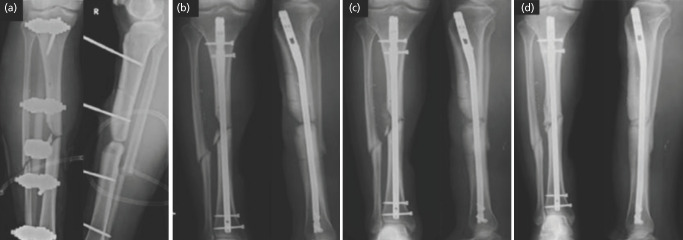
(a) Clinical radiographs of a patient with a Gustilo 3C open tibia fracture that underwent intra-medullary nailing and a pedicled hemisoleus flap. (b) This was complicated by non-union and (c) underwent bone grafting with fibrin glue (d) with subsequent union at 28 weeks.
